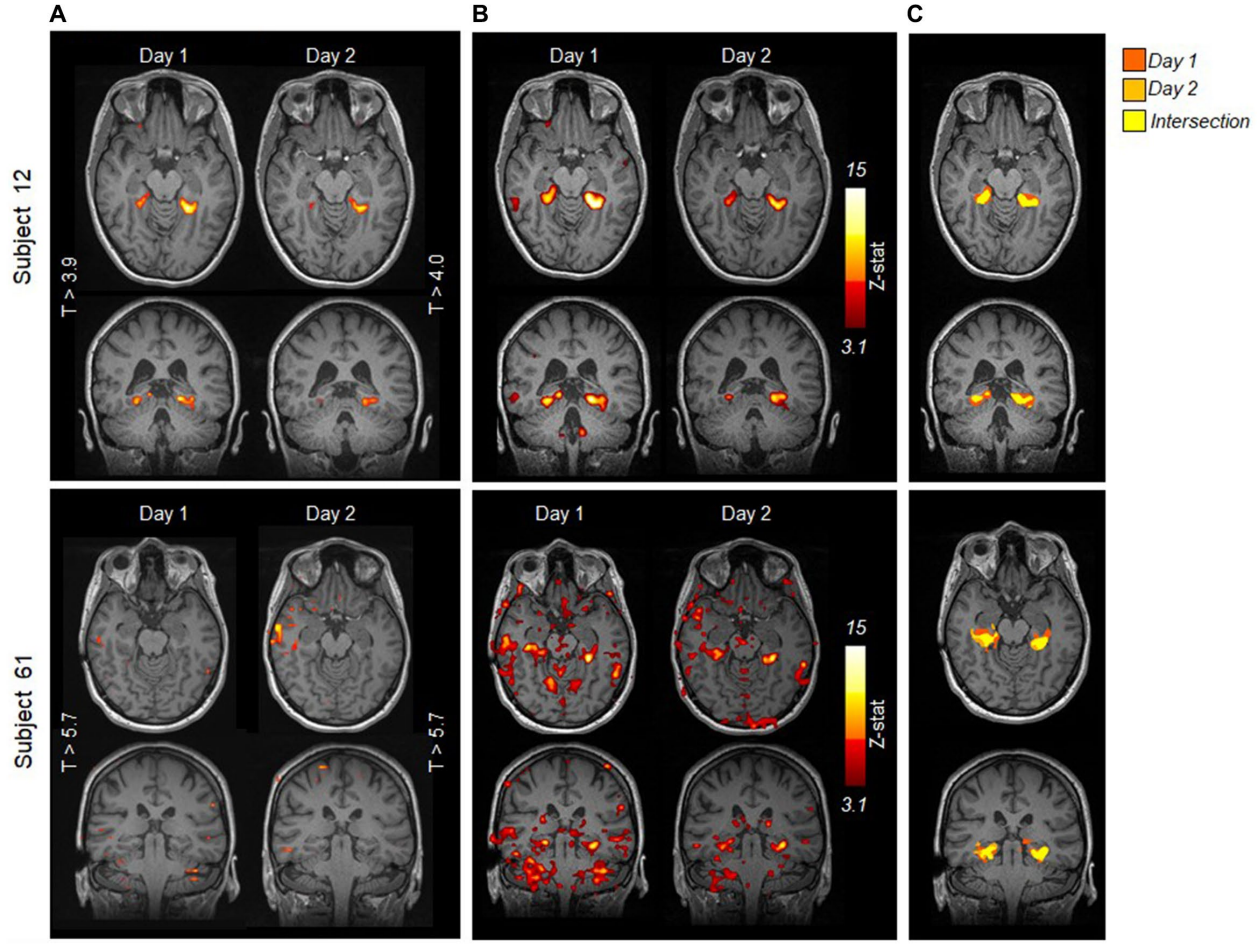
Comparison of statistical activation maps evoked by HTW task obtained with (A) clinical syngo.via analysis and (B) FSL validation analysis for two example episodes; a patient for whom the clinical analysis showed reproducible pPHG activity (top) and a patient for whom the clinical analysis fails to reveal any significant activation within pPHG in either day (bottom). Maps are overlaid onto T1-weighted anatomical MPRAGE from each day. (C) Comparison of FSL activation maps within pPHG probabilistic mask for each day on the patient’s reference anatomical volume. Color scale indicates activation from day 1 only (red), day 2 (orange) or both days (yellow).
